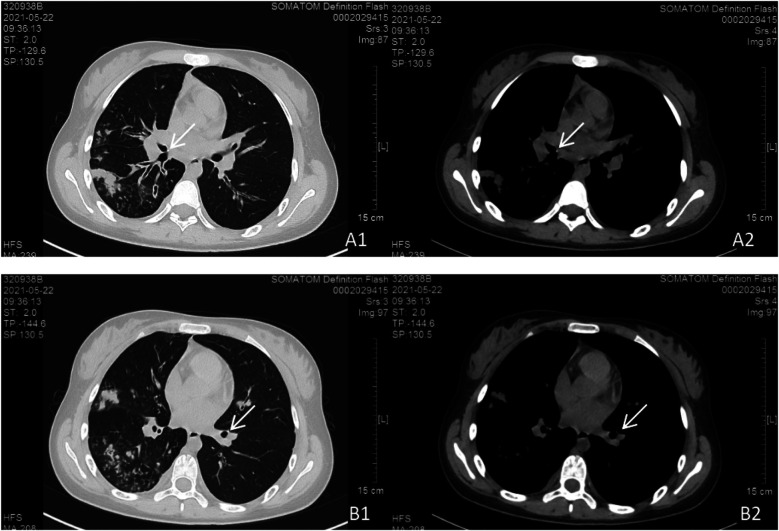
Chest CT scan findings. Bilateral pulmonary lesions were observed, with more extensive involvement in the right lung. (A1, A2) Parietal nodules adjacent to the right middle bronchus. (B1, B2) Parietal nodules adjacent to the basal segment of the left lower lobe.
